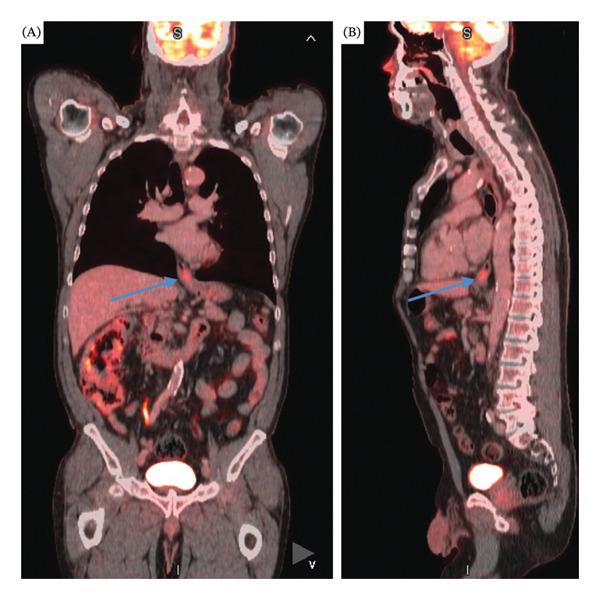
Initial positron emission tomography (PET) scan showing increased fluorodeoxyglucose‐^18^F (FDG) activity in the distal esophagus in the (A) axial and (B) sagittal planes as indicated by the blue arrow. This finding prompted an esophagogastroduodenoscopy (EGD).
